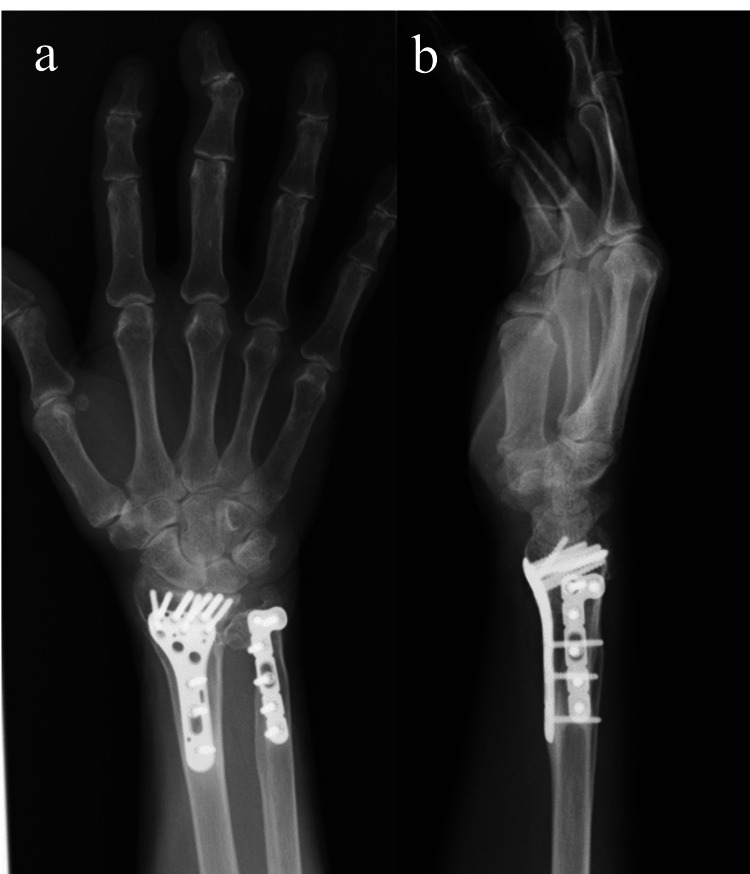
Posteroanterior (a) and lateral (b) radiographs after plate fixation for distal radius and ulna fractures. Bony union has been achieved; however, osteoarthritic changes of the distal radioulnar joint (DRUJ) are present.
